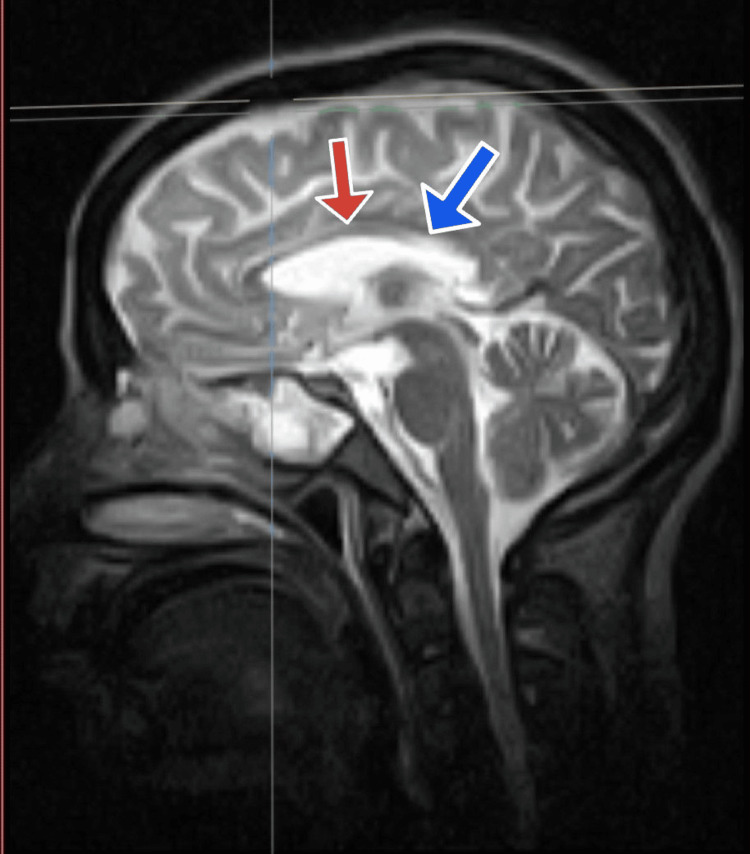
An MRI image of the brain with the red arrow shows the thinning of the corpus callosum while the blue arrow shows increased signals in the splenium
